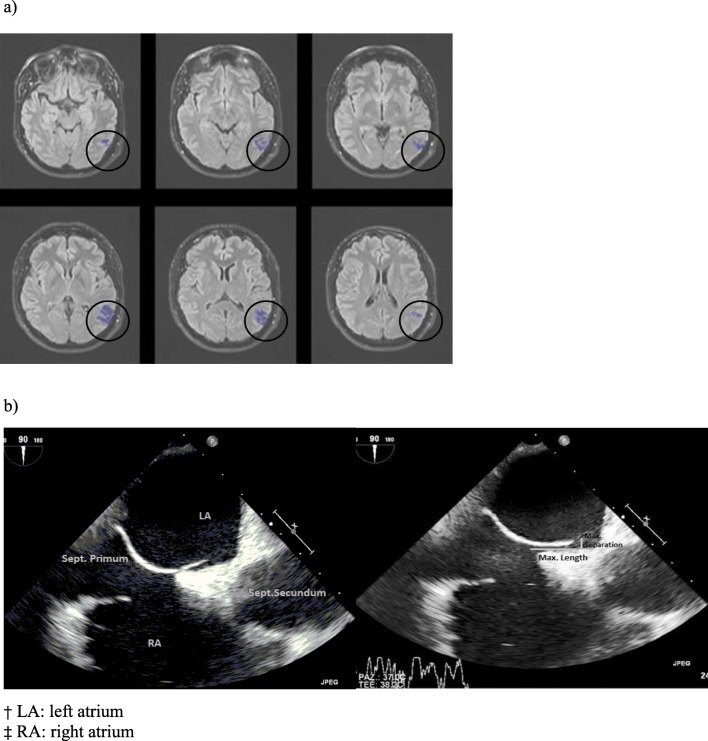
a Magnetic resonance images: ischemic lesion volume, FLAIR sequences; b TEE images: patent foramen ovale dimensions. † LA: left atrium, ‡ RA: right atrium
